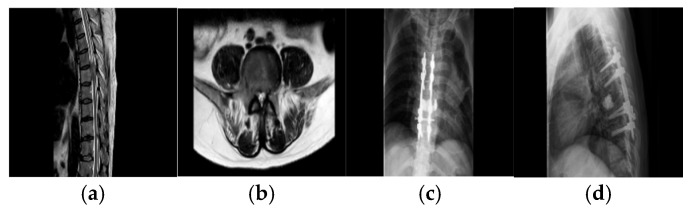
(a,b): Preoperative MRI demonstrates tumor involvement of the T7 vertebral body. (c,d): Postoperative anteroposterior and lateral radiographs at 1 week show satisfactory recovery.
